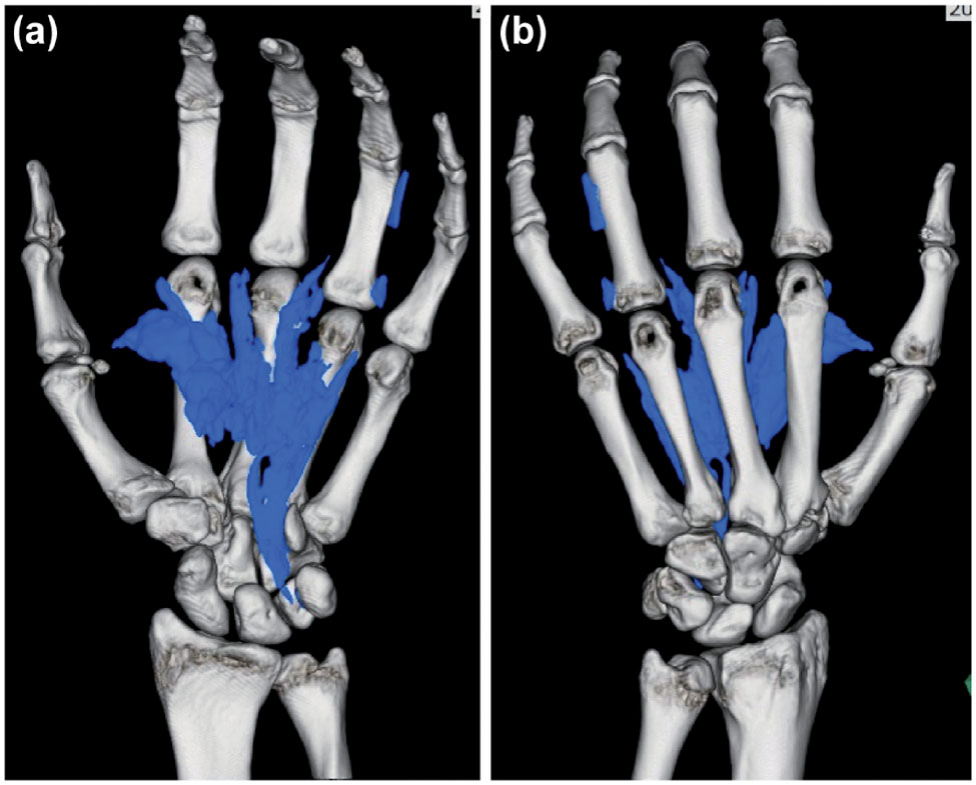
Computed tomography images acquired after the first operation show paint in the thenar space and the index, middle, and ring fingers.
